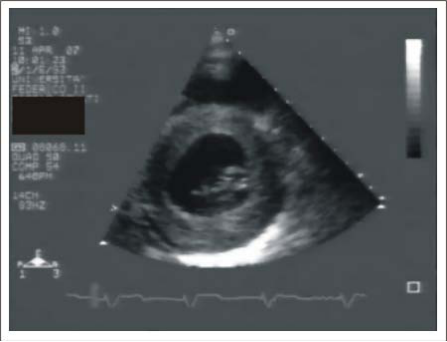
Patient with definite diagnosis of mithocondropathy done by neurologists. Echocardiography shows mild symmetric hypertrophy with mild reduction of left ventricular ejection fraction. During follow-up patient developed severe grade of AV block needing pace-maker and severe reduction of ejection fraction needing resynchronization.
